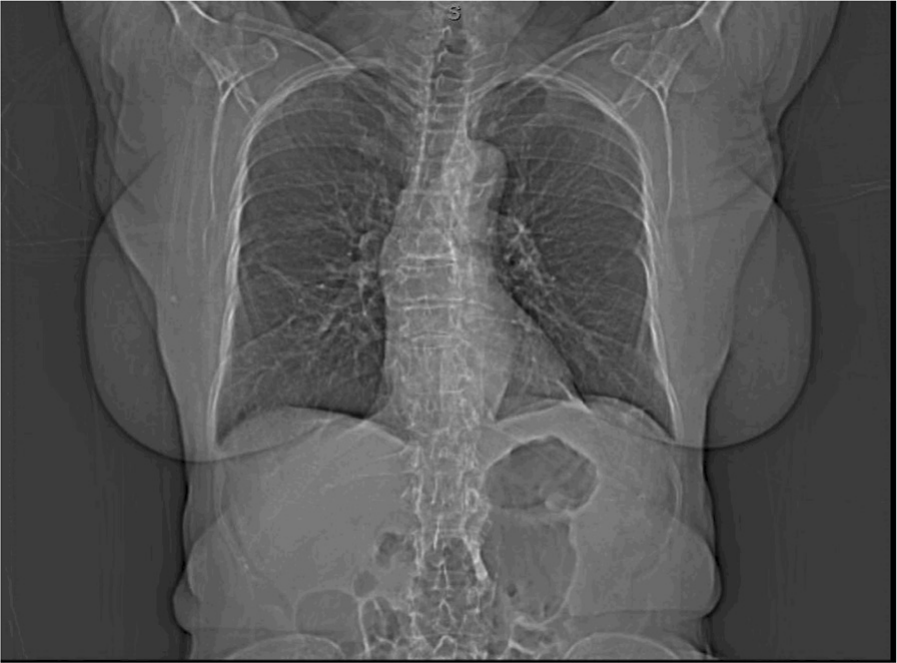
Coronal view of a computed tomography scan of the chest.
